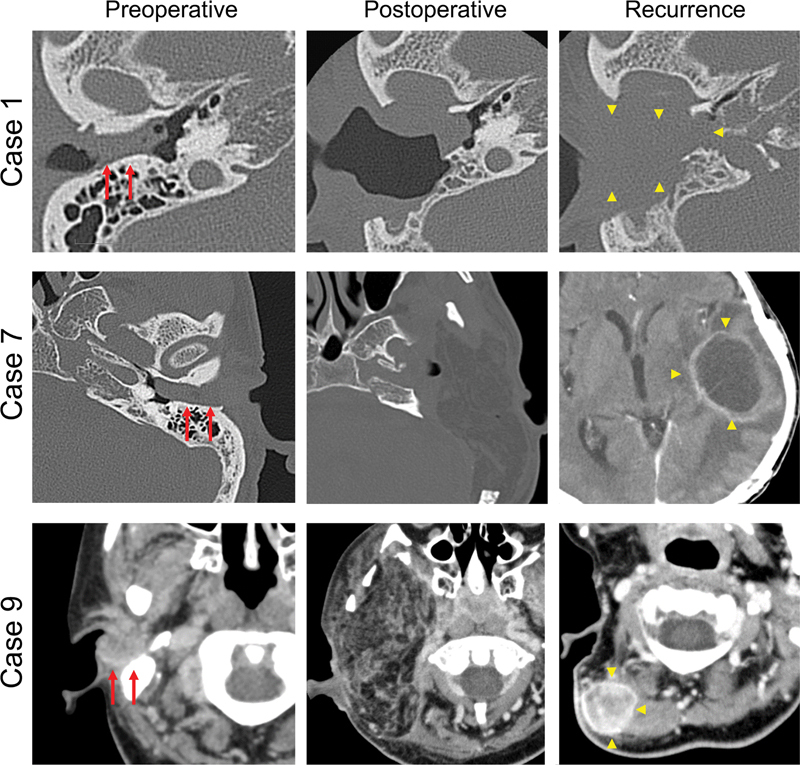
Representative computed tomography images of the three surgical patients: preoperative, postoperative, and at the time of recurrence.
